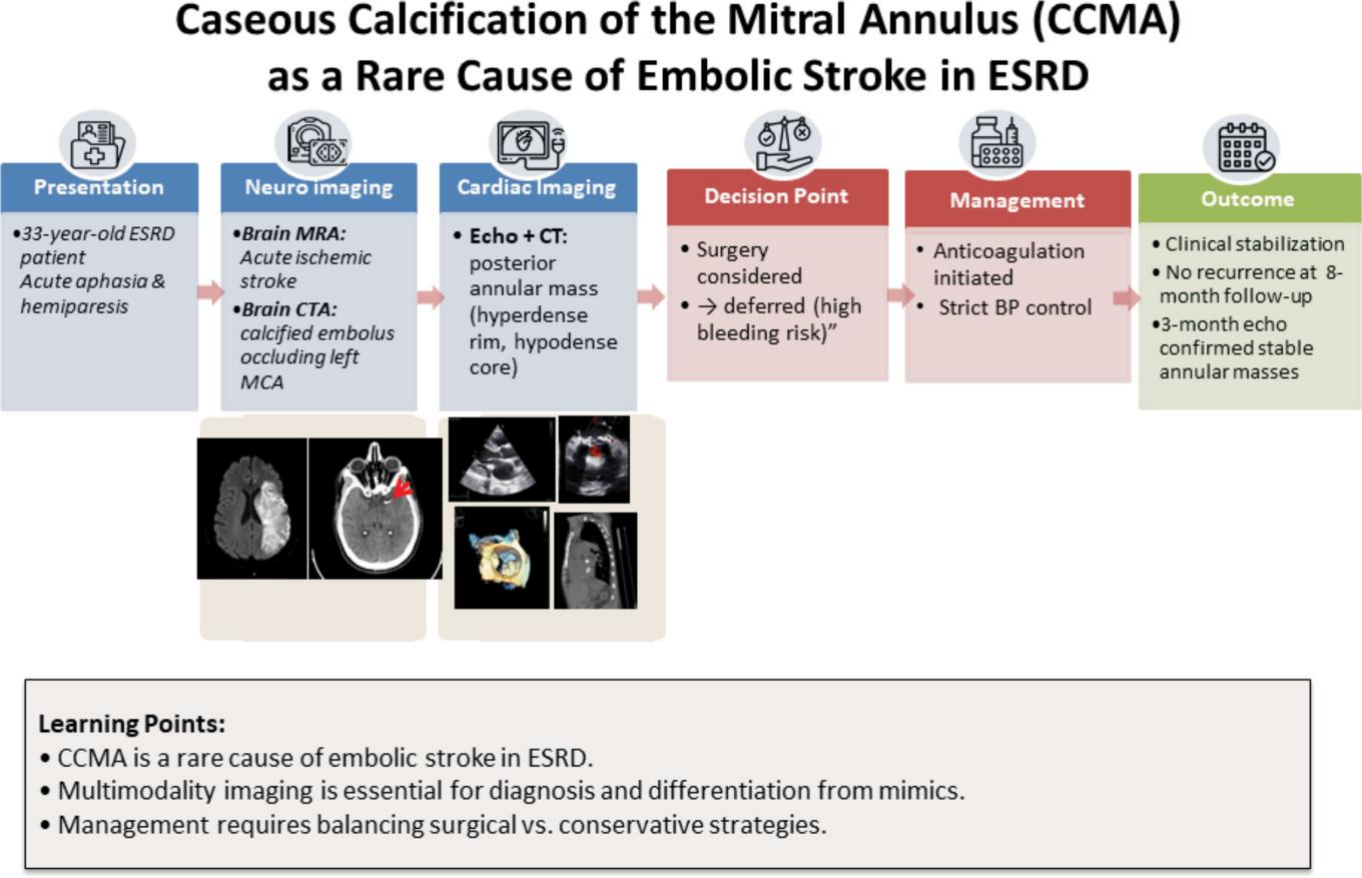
Timeline of clinical events in a 33-year-old dialysis patient presenting with acute ischaemic stroke. Brain MRA demonstrated infarction, and CTA revealed a calcified embolus in the MCA. Echocardiography and cardiac CT confirmed caseous calcification of the mitral annulus as the embolic source. A multidisciplinary team deferred surgery and opted for delayed anticoagulation with strict blood pressure control.
